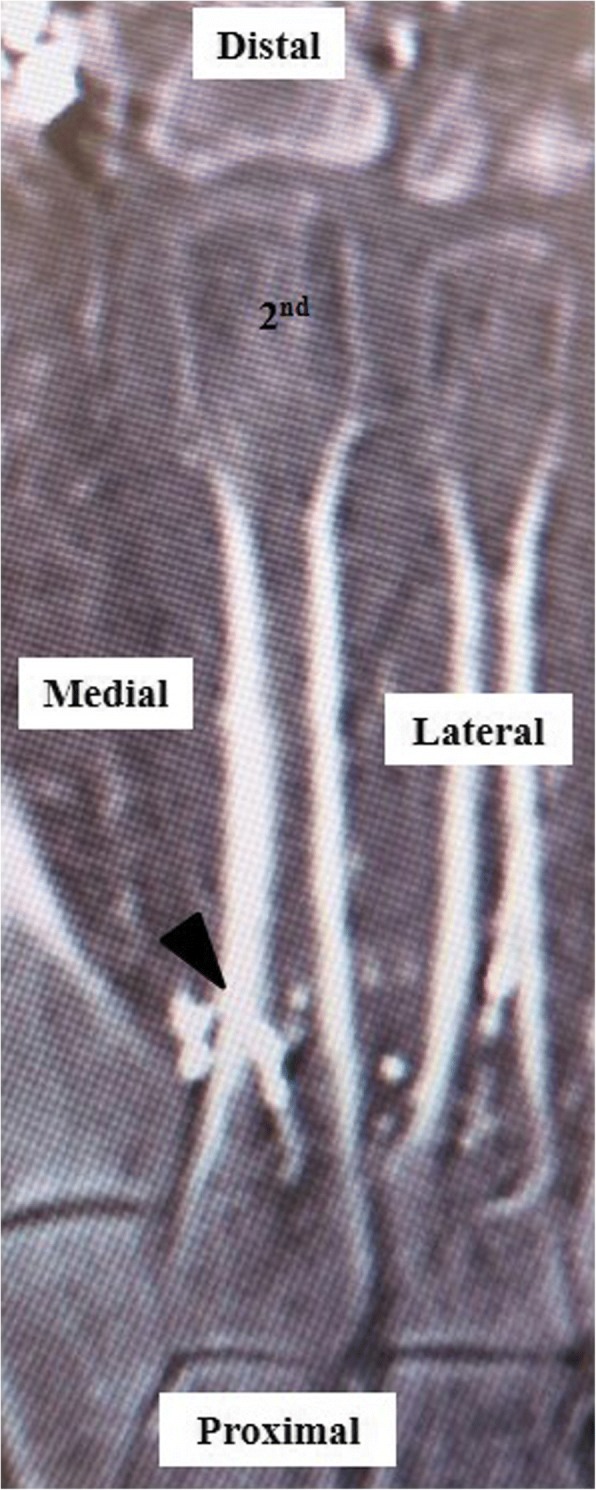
Enhanced CT showing the nutrient artery entering the lesser metatarsal near the mid-point. The nutrient artery enters the lesser metatarsal near the mid-point from a distal direction laterally and obliquely in the coronal plane. The arrow head indicates the nutrient artery
